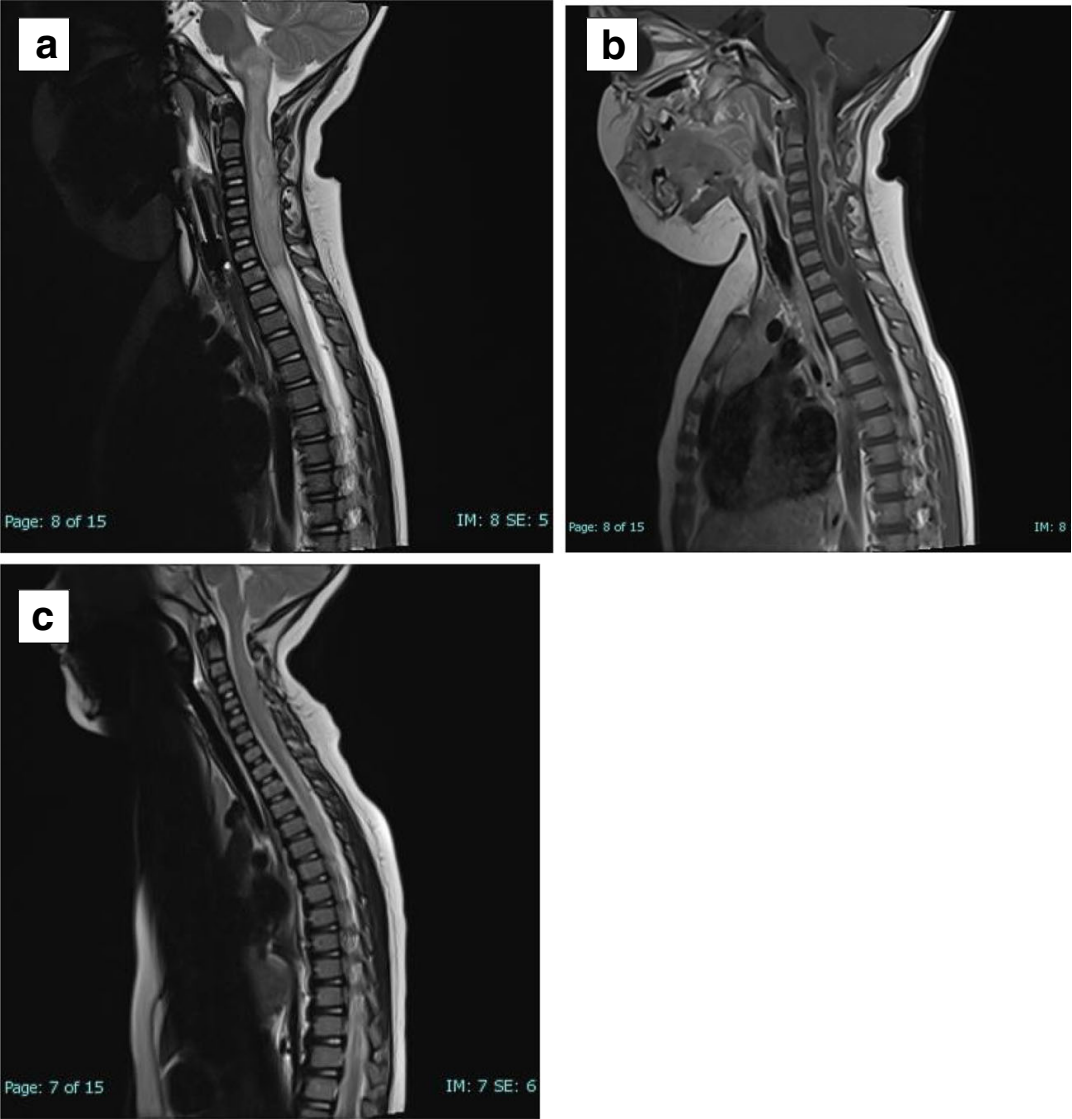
Preoperative sagittal MRI of the spine.: (a) T2-weighted image showing oedema of the pons extending down to T1 with an associated intradural lesion at C4.. (b) Preoperative T1-weighted MRI with contrast showing an extensive intramedullary abscess. (c) Post-operative T2-weighted MRI of the spine at 9 months showing resolution of the abscess and oedema
